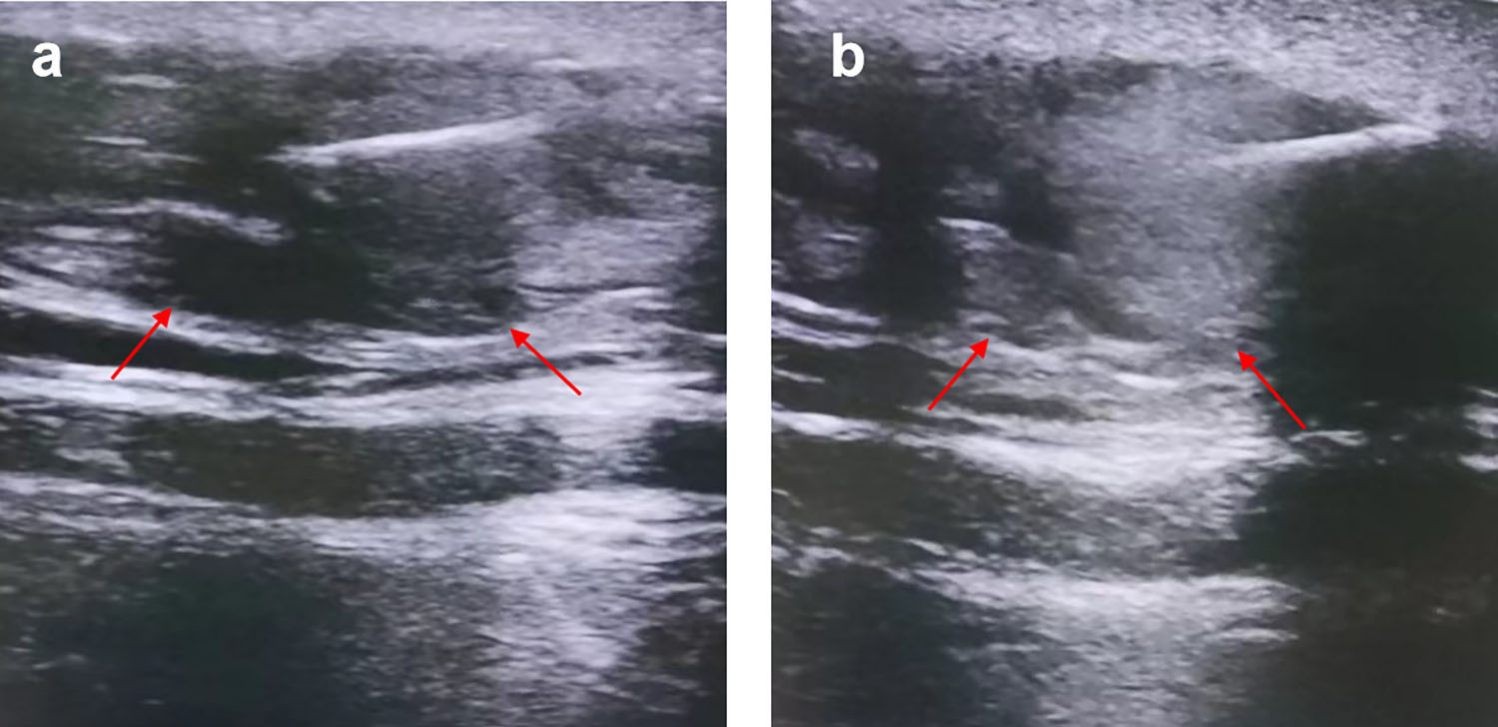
An intraoperative ultrasound image of lipoma ablation; (left) the red arrow shows a low-density lipoma before ablation; (right) the red arrow shows that the density of lipoma after ablation is higher than before
